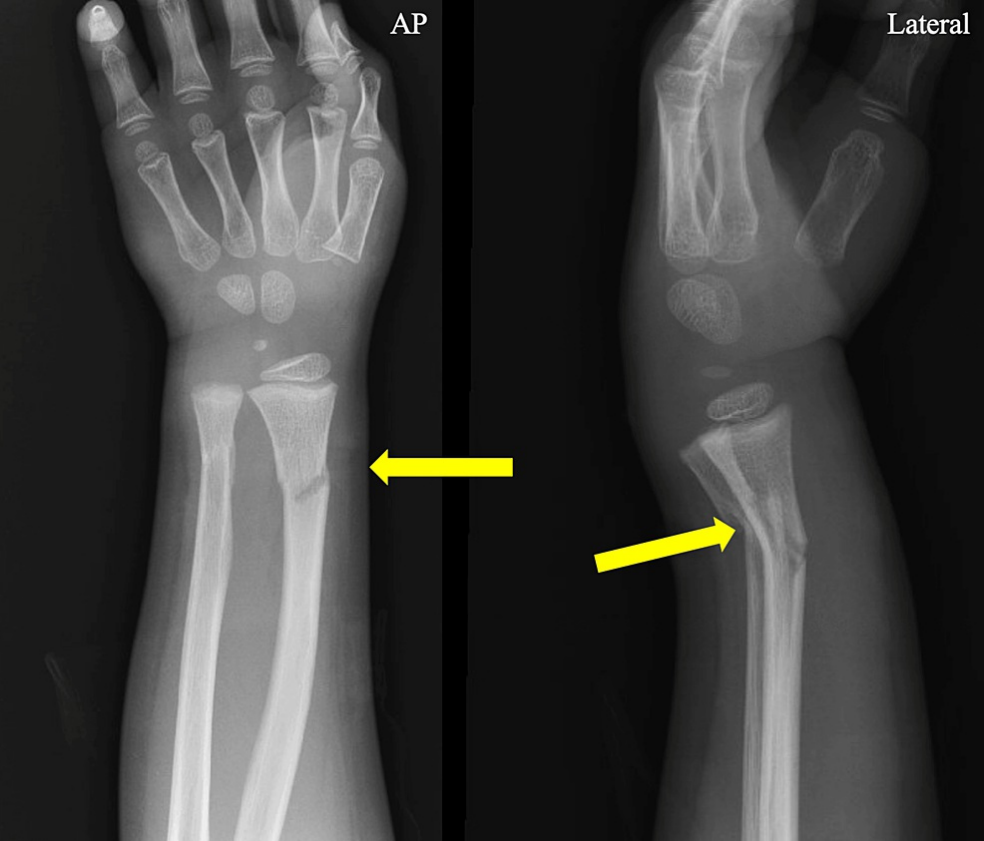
AP and lateral X-ray imaging of the left wrist The arrows point to dorsally angulated, extra-articular distal radius, and ulna fractures
